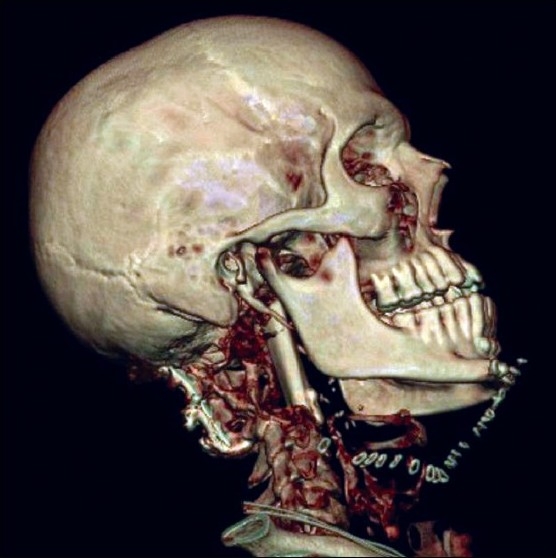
Postoperative three-dimensional CT image
